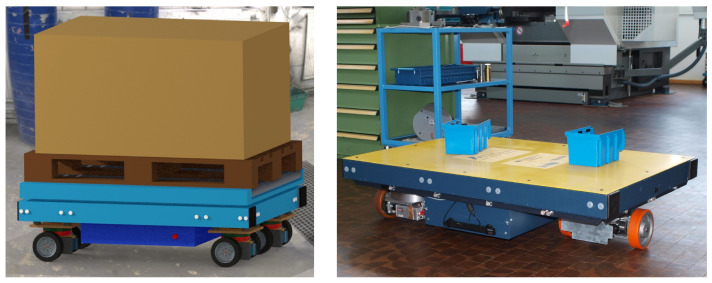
Redundant ultrasonic sensors at the side of the AGV.
